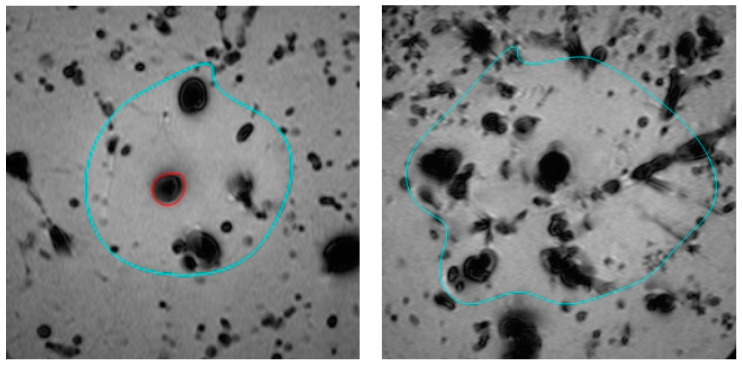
Tissue carbonisation (red) and ablation margin (turquoise) during laser ablation visualised in postprocedural T1-weighted MRI of the liver: 0.9% saline cooling fluid (left) and a mixture of 0.9% saline and distilled water (right).
